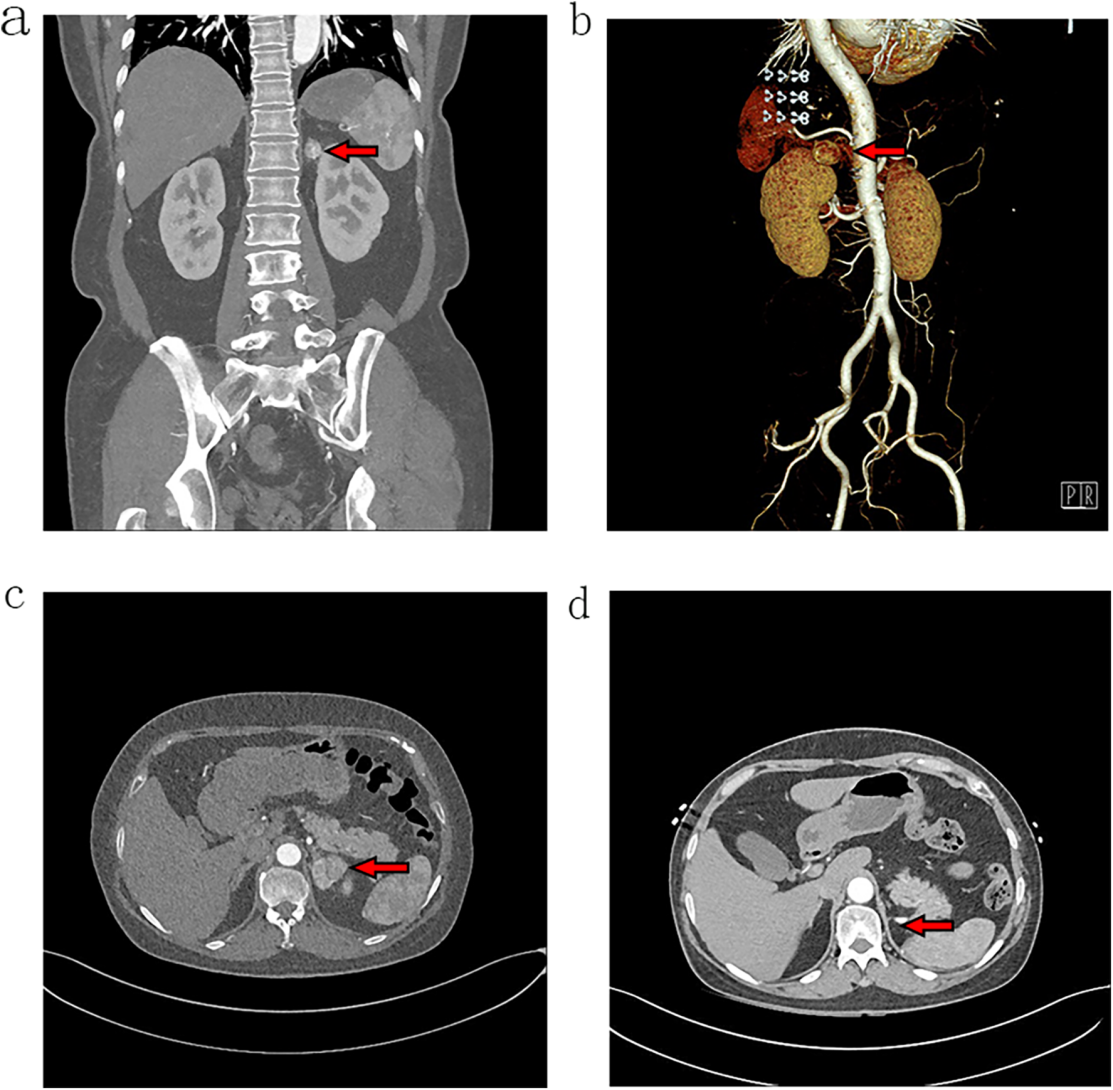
Abdominal CT images with contrast enhancement. Coronal views (a,b) and a cross-sectional view (c) reveal a soft tissue mass in the left adrenal region, characterized by mild and uneven enhancement. (d) The follow-up cross-sectional view at 6 months postoperatively hows no evidence of tumor recurrence in the left adrenal region.
